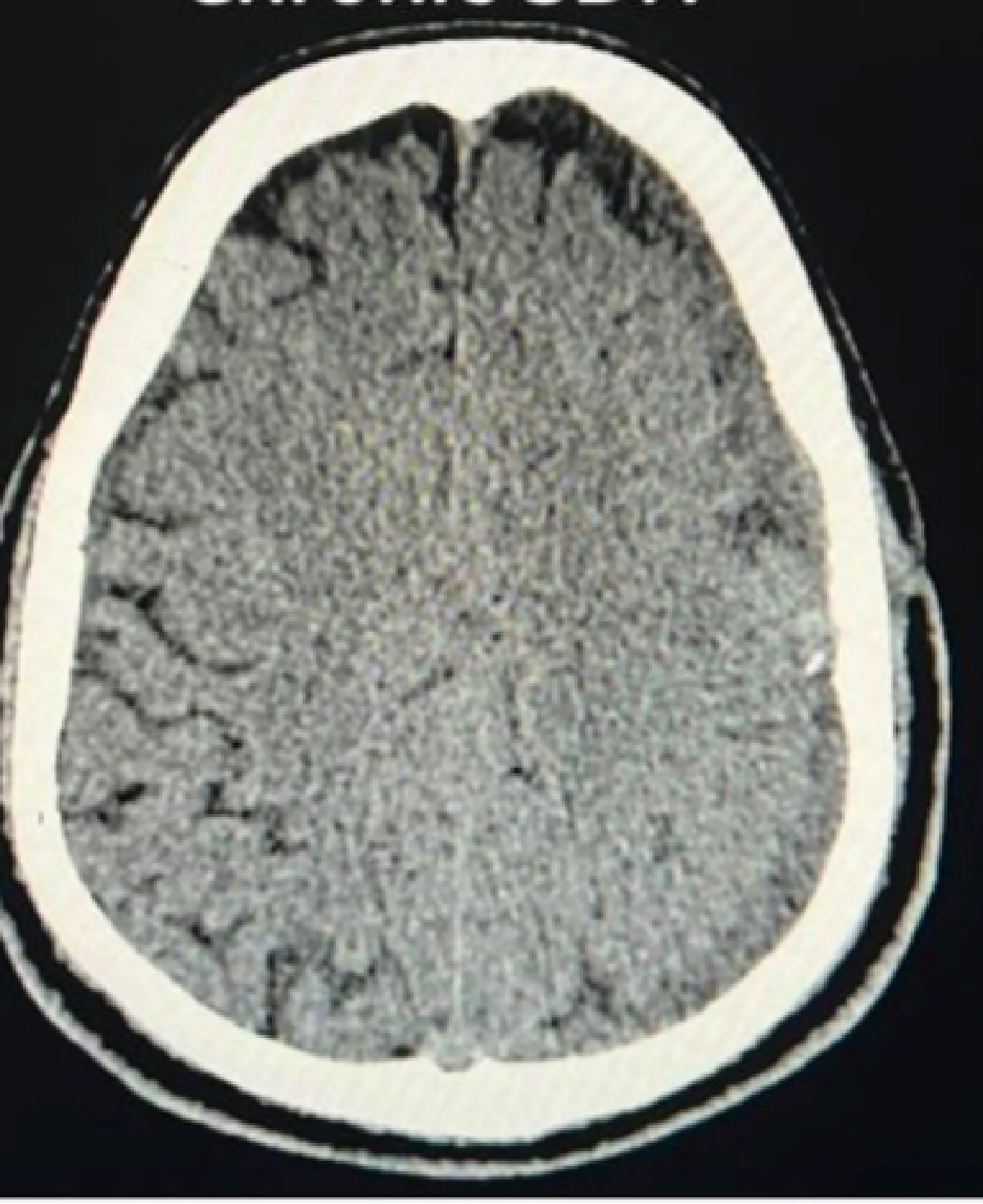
CT scan showing reaccumulation of blood in the subdural space after burr hole surgery.
